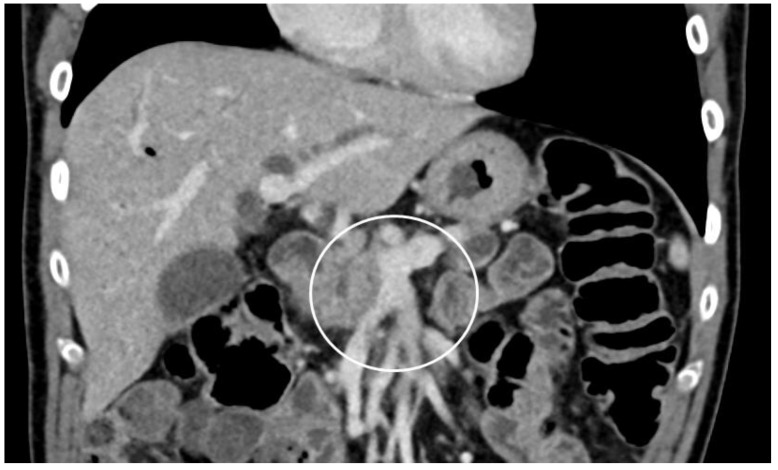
Anatomical borderline resectability, contrast enhanced CT scan, and coronary reformatting. Pancreatic head cancer with contact to superior mesenteric vein/portal vein confluence (white circle), vascular reconstruction technically possible.
